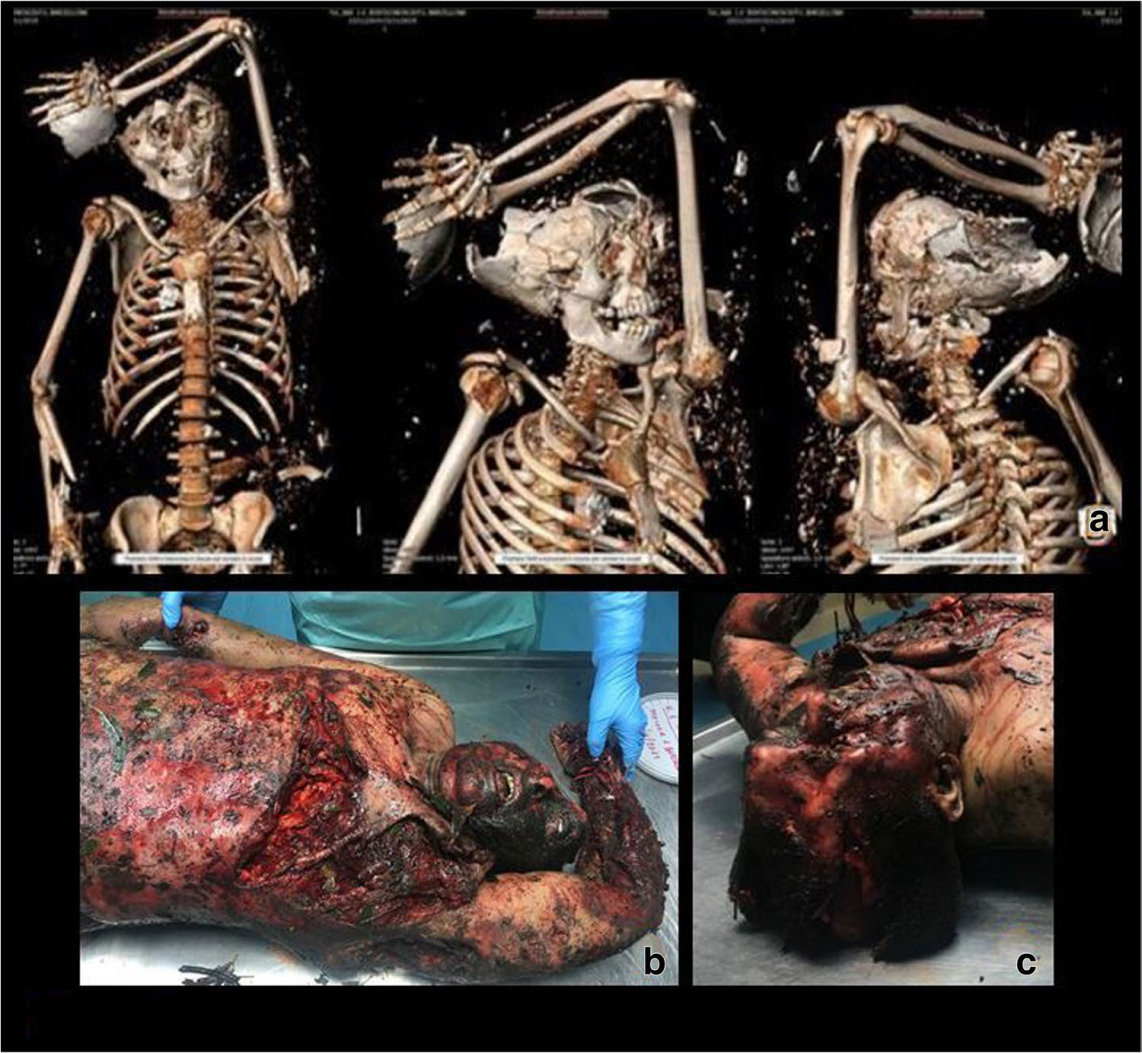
Subject no. 1: 3D CT scan (a) and details of the lesions detected on external inspection (b and c)
